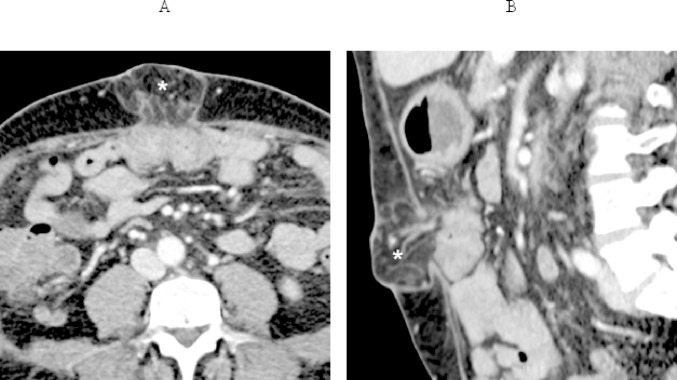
Axial (A) and sagittal (B) CT images demonstrate a true umbilical hernia with a peritoneal-lined sac containing omentum (*) protruding through a narrow fascial neck. The herniated omentum can be distinguished from preperitoneal fat by its direct intracavitary continuity, particularly by tracing its vascular structures as they remain contiguous with the intra-abdominal omental vessels. In contrast to the umbilical canal, embryologic remnants such as the median umbilical ligament and the ligamentum teres are not identifiable within the hernia sac
